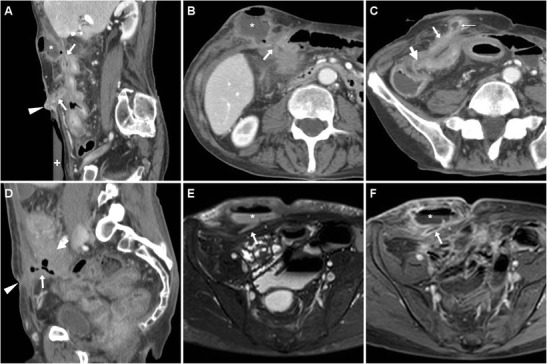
Two cases of spontaneous ECF complicated by abscess collections in chronic inflammatory bowel diseases. A 77-year-old man with indeterminate colitis treated by proctocolectomy and definitive ileostomy developed two ECFs, one in right hypochondrium with a sizeable superficial abscess (* in A, B) communicating (arrows) with a jejunal loop; the second, longer and more distal ECF (arrow in C) originated from the thickened ileum (thick arrow) and included a smaller subcutaneous abscess with inflamed mucosa (thin arrow). In a 59-year-old woman with fistulising CD, the ECF corresponded at CT (D) to a gas-filled track (arrow) arising from the diseased ileocolonic anastomosis (thick arrow) and reaching a subtle depressed EFO (arrowhead). Follow-up MRI including T2- (E) and post-contrast fat-saturated T1-weighted (F) showed development of a large abscess (*) with fluid content and enhancing wall along the ECF (arrows); definitive surgical treatment required extensive adhesiolysis, resection of rectum and of diseased perianastomotic bowel
